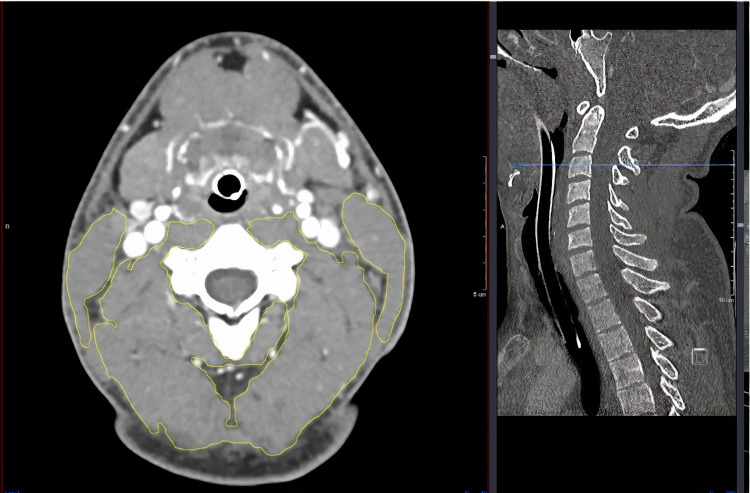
Axial CT image of total skeletal muscle (left) delineated in yellow at the level of the C3 vertebra
